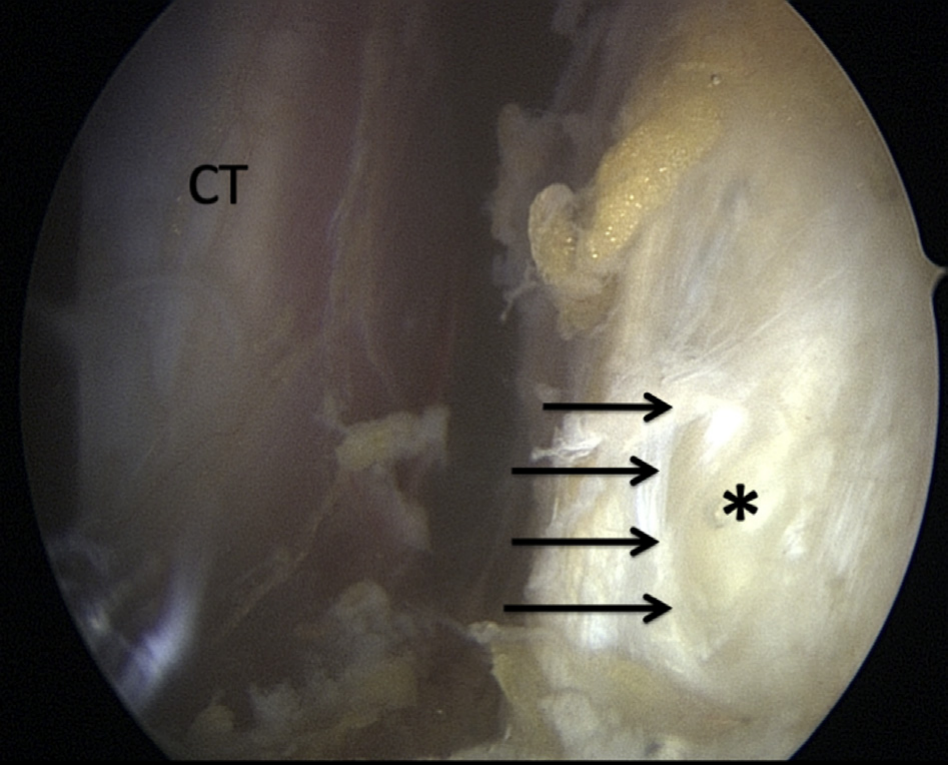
Subcoracoid space of a left shoulder, ASL viewing portal. After LHB tenotomy, the bicipital sheath (black arrows) is opened laterally and the LHB (∗) is found. (ASL, anterosuperolateral; CT, conjoint tendon; LHB, long head of the biceps.)
